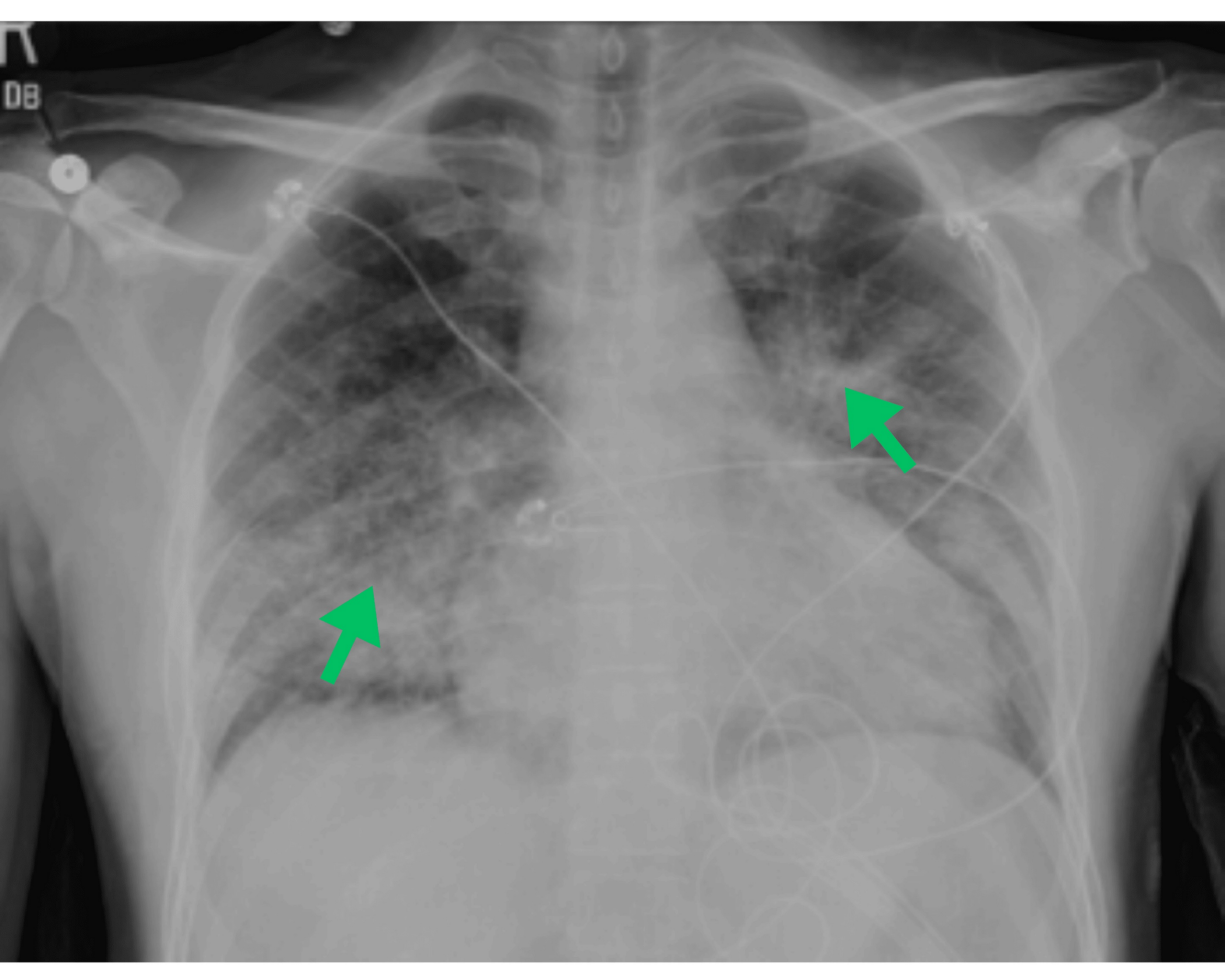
Initial chest X-ray demonstrating heterogeneous bilateral opacities with a reticulonodular pattern, along with perihilar interstitial markings and no signs of infiltration.
